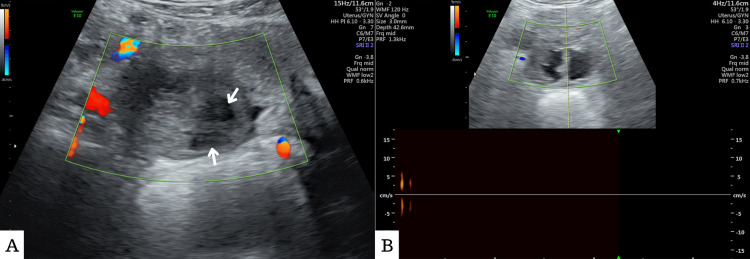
Ultrasound scan A. Two cystic spaces (white arrows) in the right ovarian tissue. B. No vascularity with color Doppler
